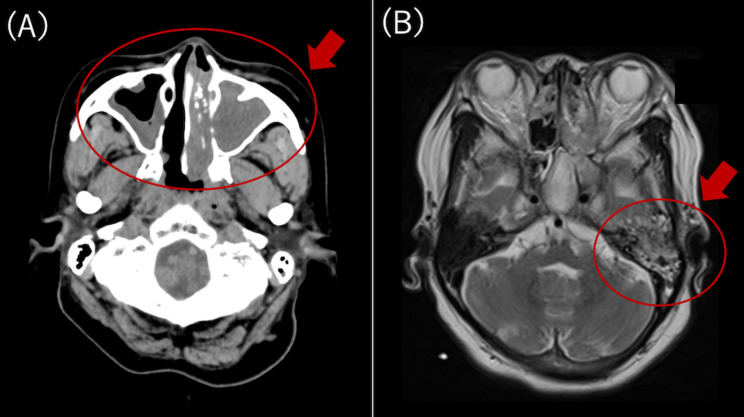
Head computed tomography(CT) and magnetic resonance imaging findings on admission. (A) Axial contrast-enhanced CT showing marked mucosal thickening and fluid retention in the paranasal sinuses, predominantly on the left side (red circles). (B) Axial T2-weighted MRI demonstrating opacification of the left tympanic cavity and mastoid air cells (red circle), consistent with left-sided otitis media. Red arrows indicate locations of major inflammatory changes
